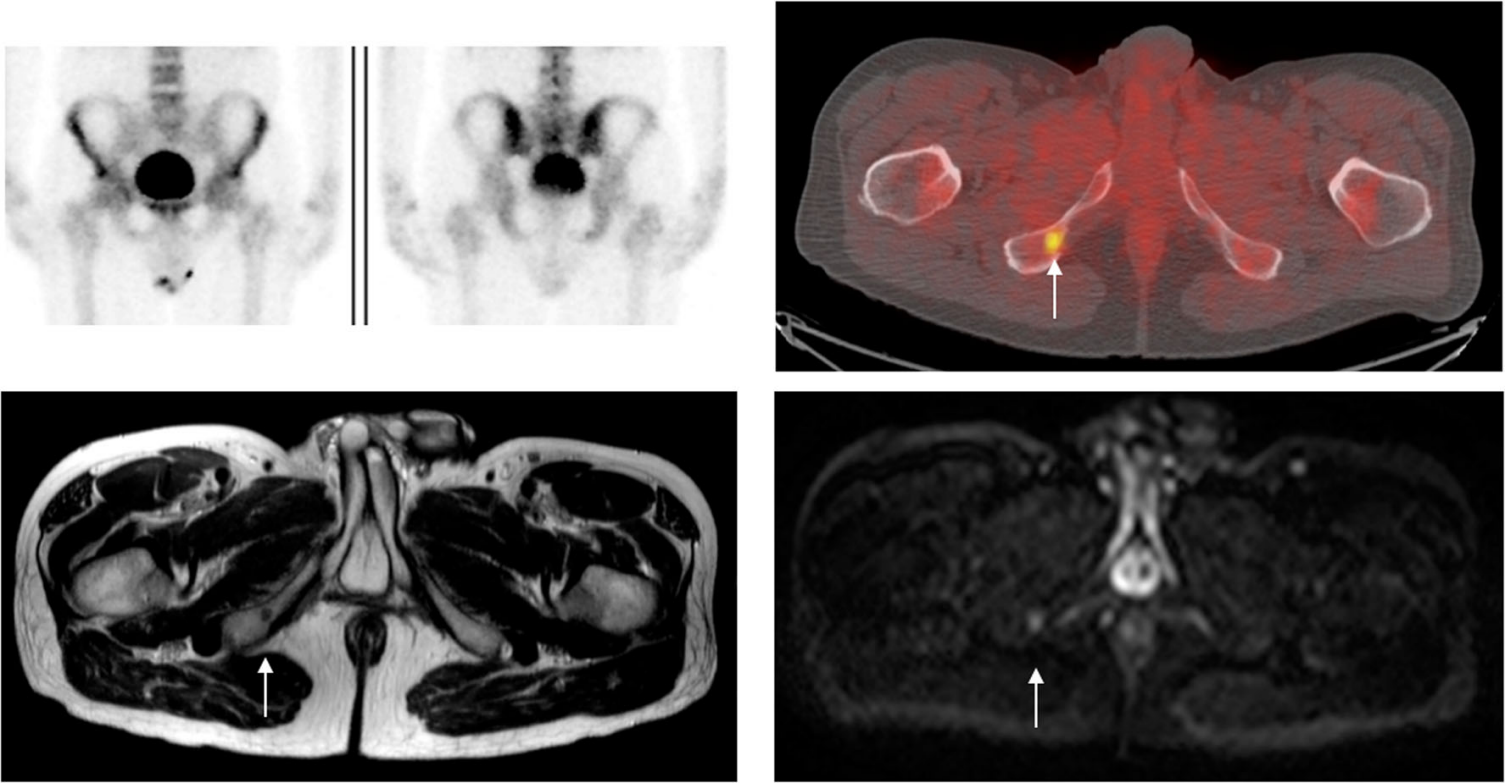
Example of discordant skeletal site in a 64-year-old man with a serum prostate-specific antigen level of 25.2. Top left: Negative ^99m^Tc bone scintigram (BS), Top right: ^18^F-choline PET/CT showing an area of increased tracer avidity at the right inferior pubic ramus consistent with a metastasis. Bottom left: Axial T2W TSE showing the lesion is of low signal intensity. Bottom right: the lesion has increased diffusion-weighted signal on *b* = 1000s/mm^2^. The lesion was considered as an example of a true positive whole-body MRI, true positive ^18^F-choline PET/CT, and false negative BS
